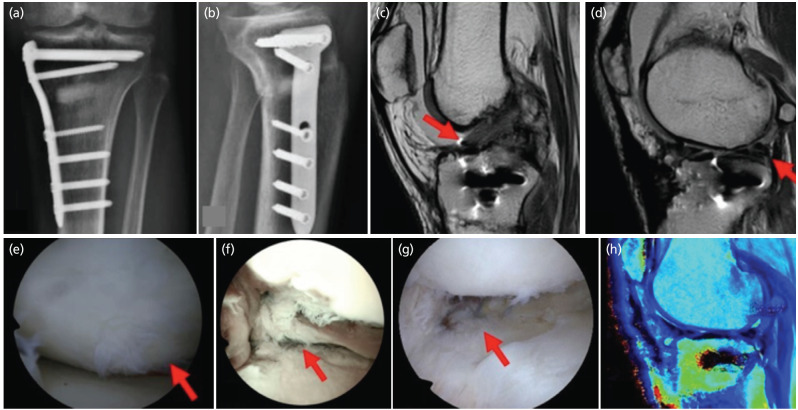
Clinical follow-up images. (a) Radiographs of the left knee four months post-operatively. Osteotomy hardware in an adequate position and evidence of bone healing. AP view. (b) Lateral view. (c) MRI after trauma with fat-suppressed T2-weighted fast spin-echo sequence. The arrow indicates the ACL injury. (d) The arrow indicates the injury to the posterior root of the transplanted meniscus. (e) Osteochondral graft healing in the lateral femoral condyle during the second surgical procedure. (f) Arthroscopic view, acute posterior root tear of the transplanted lateral meniscus during the second surgical procedure. (g) Sutured posterior root lesion of the lateral meniscus. (h) Post-operative sagittal view with MRI T2-mapping sequence, without an overload of the lateral compartment.
